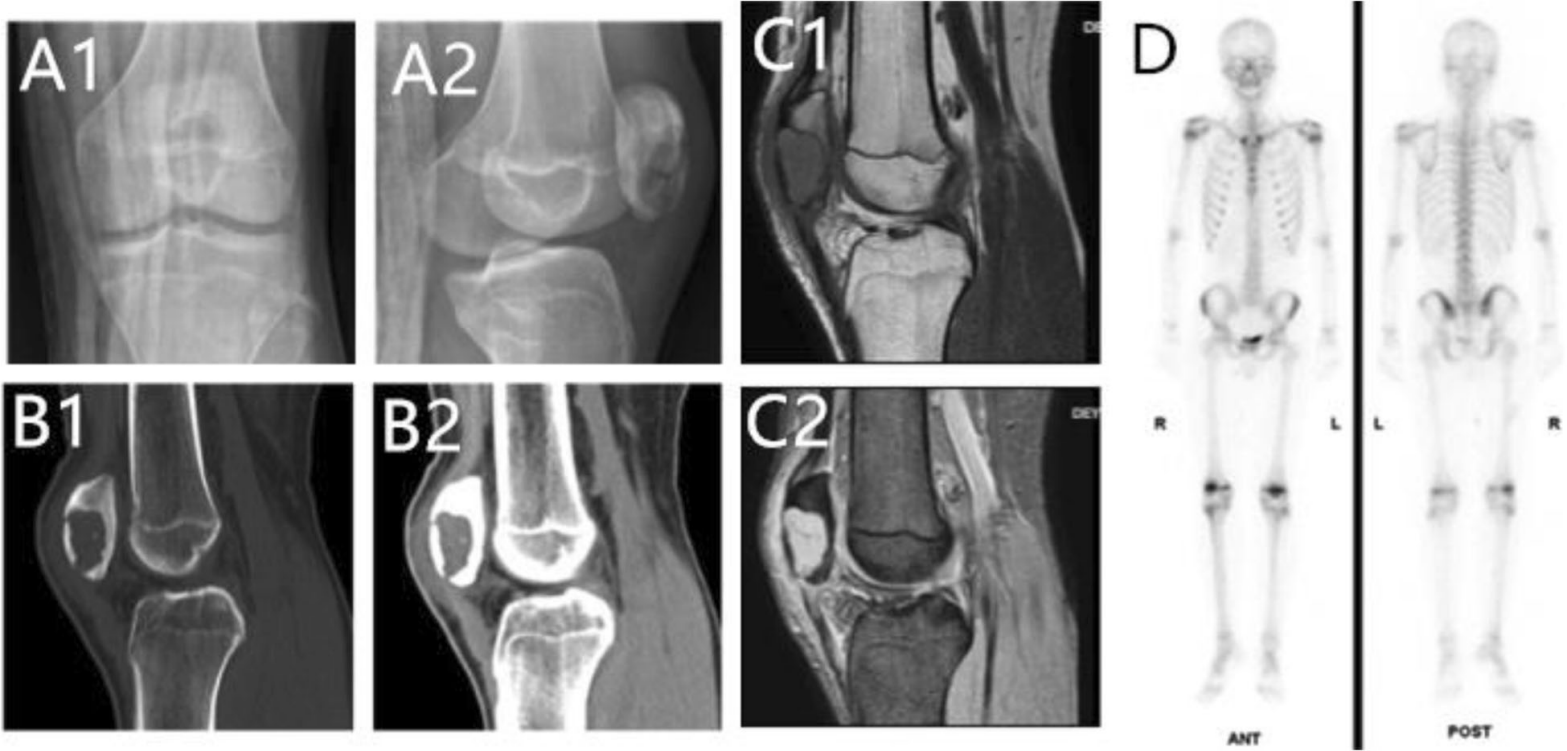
Images of the patient who had pathological fracture of his left patella. A1, A2 Anteroposterior and lateral views showed a well-demarcated, eccentric and osteolytic lesion with pathological fracture of the left patella. B1, B2 CT scan revealed a stage 2 patella lesion (1.5 × 2 × 3 cm) with a thinned cortex, a clear boundary and pathological fracture. Calcifications inside the lesion are shown very well in B2. The lesion did not break through the articular cartilage and did not invade the adjacent femur or tibia. C1, C2 MRI showed that the lesion appeared as slightly intense on T1-weighted images (C1, through the knee in sagittal planes) and intense on T2-weighted images (C2, through the knee in sagittal planes). The soft tissue showed edema at the anterior of the patella. D Whole-body bone scan of the patient. A bone scan showed moderate accumulation of the radiopharmaceutical agent in the left and right patella without strong accumulation in other parts of the body
